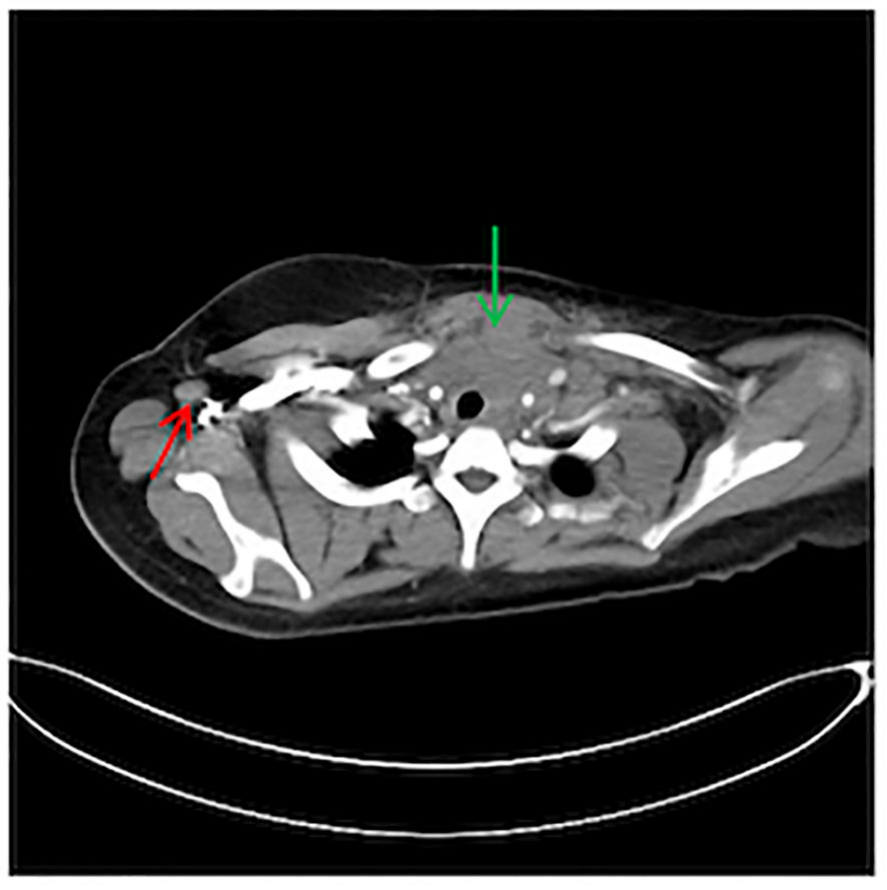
Chest computed tomography (CT) showing an anterior mediastinal mass (Green) and right axillary lymph node enlargement (Red).
